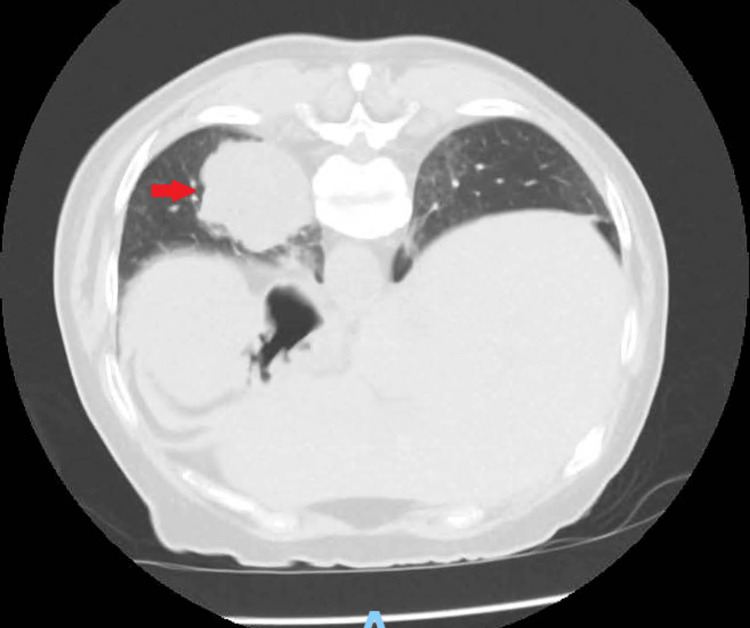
CT scan on September 27, 2022, showing mass of 5 x 4 cm in lower left lobe (red arrow) This image corresponds to the primary tumor before being operated on.
